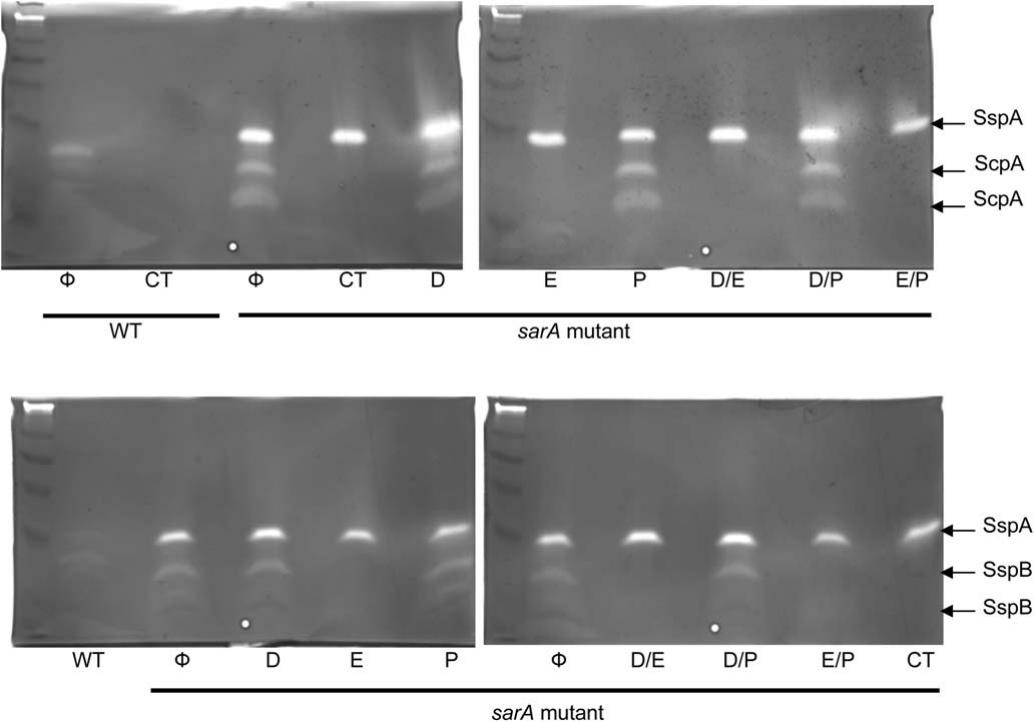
Zymogram analysis of protease production in the presence of protease inhibitors. Analysis of the production of specific proteases was assessed using casein (upper panel) and gelatin gels (lower panel) in UAMS-1 (WT) and its UAMS-929 *sarA* mutant. Analysis was done after growth in biofilm medium in the absence of any inhibitor (Φ), the presence of the inhibitor cocktail (CT), or the presence of each inhibitor alone and in combination with each other. Inhibitor designations are the same as in the legend for Fig. 10. The presumed identity of individual proteases is indicated to the right. In the case of SspA, this was confirmed by analysis of the corresponding *sarA/sspA* mutant (data not shown). In the case of ScpA and SspB, identification is presumptive based on their identity as cysteine proteases, inhibition of both by E-64, and the fact that ScpA has greater activity than SspB on casein while the opposite is true on gelatin (Dr. Jan Potempa, personal communication).
